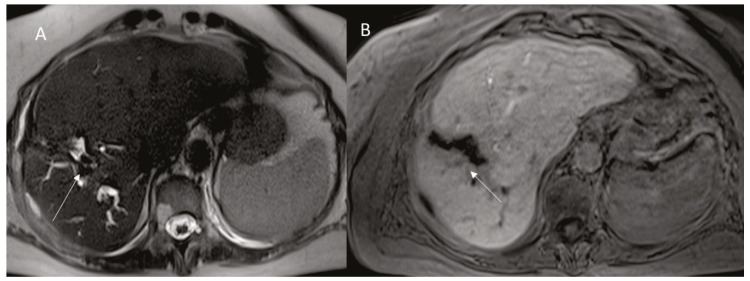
The same patient of Figure 2, MRI assessment ((A): HASTE T2-W sequence, (B): EOB-Phase of contrast study) after 2-month, arrow shows biloma.
